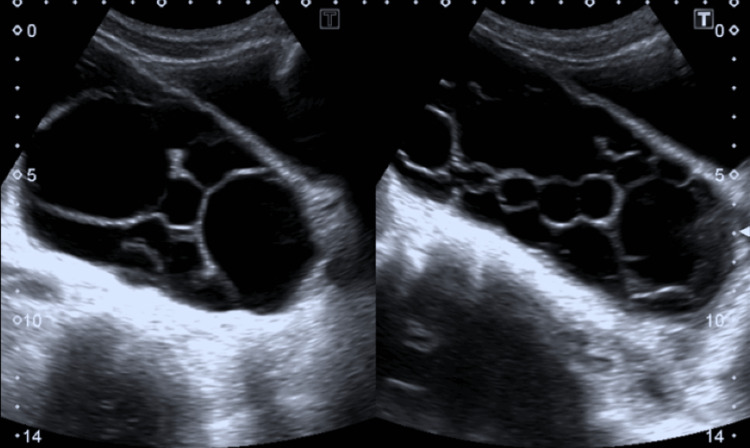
Pelvic ultrasound showing multiple cysts in the pelvic cavity.
